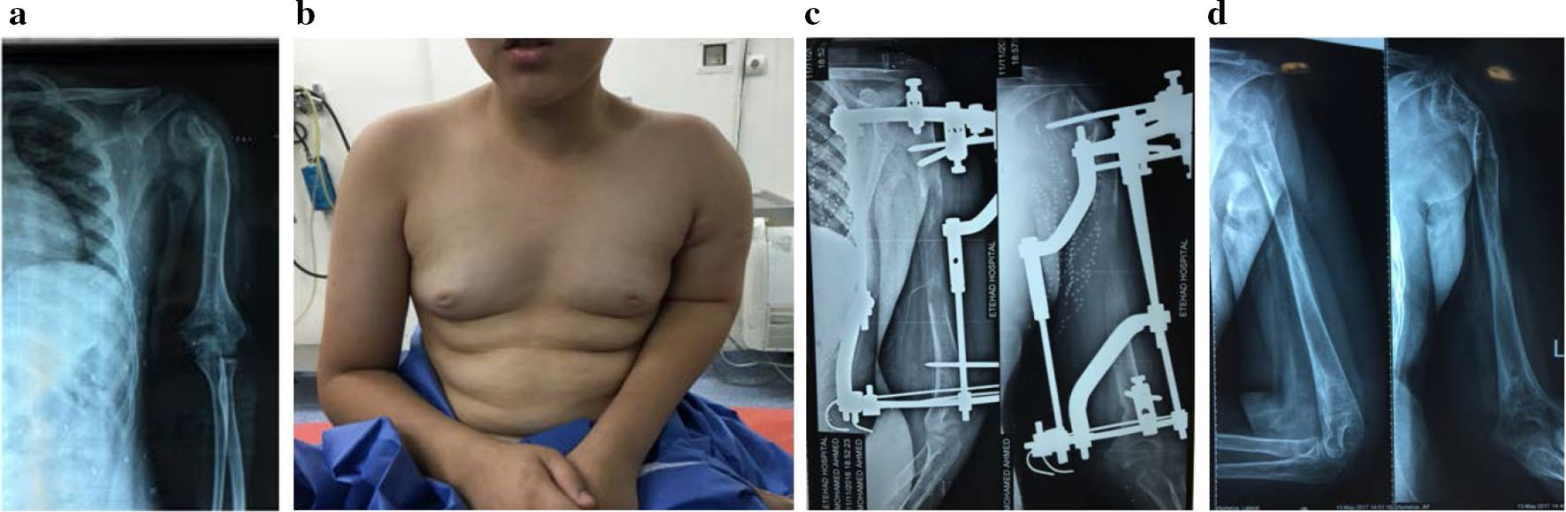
a A 12-year-old boy with 10-cm post-traumatic humeral shortening. b Clinical photo showing left humeral shortening. c Plain X-ray at the end of distraction. d Plain X-ray after fixator removal
